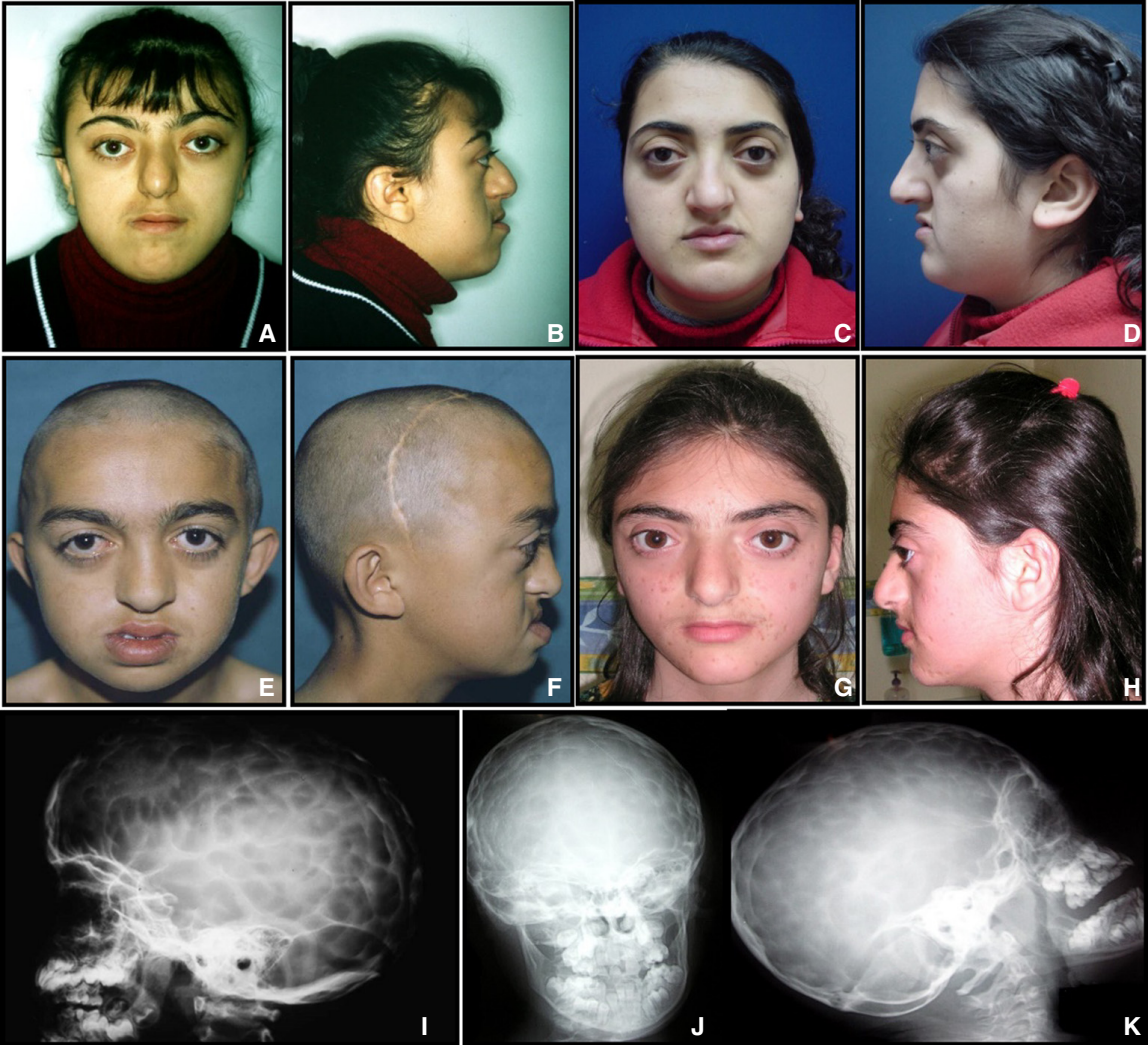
Craniosynostosis phenotypes linked to *IL11RA* mutations. (A-H) Facial views of representative cases of the Turkish CRS1 family with Crouzon-like craniosynostosis. (A and B) Subject IV:5. Facial appearance at 17 years. (C and D) Subject IV:11 at 16 years old. (E and F) Affected individual of the CRS3 family carrying the p.Arg292* mutation at approximately 9 years of age. All three subjects were treated by craniotomy at an early age. (G and H) Affected individual of the CRS4 family with p.Tyr232* mutation at 12 years of age. (I-K) Skull X-ray with increased digital markings and maxillary hypoplasia present in CRS3.
